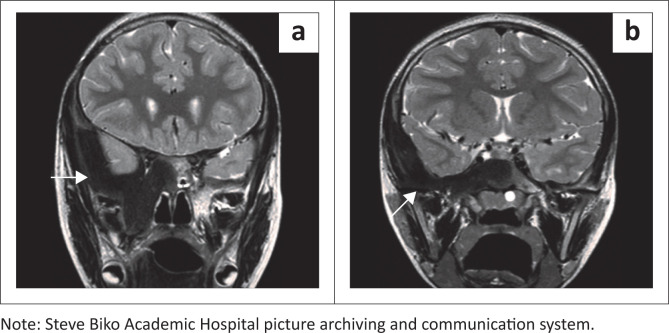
Coronal T2-weighted MRI images (a, b) confirm focal unilateral involvement of the spheno-temporal bone with homogenous hypointensity (white arrows). Associated extension into the pituitary fossa is demonstrated with minimal cranial displacement of the hypothalamic-pituitary axis. The hypothalamic-pituitary axis was otherwise normal.
